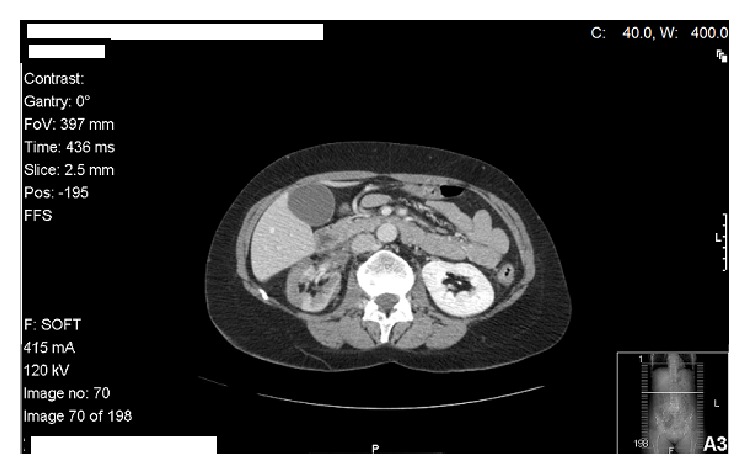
Abdominal Computer Tomography (CT) scan showing diffuse reduction in the parenchymal thickness of the right kidney and multiloculated fluid collection of about 10 centimetres (cm), involving the anterior part and the upper pole of the right kidney and the ipsilateral renal artery.
